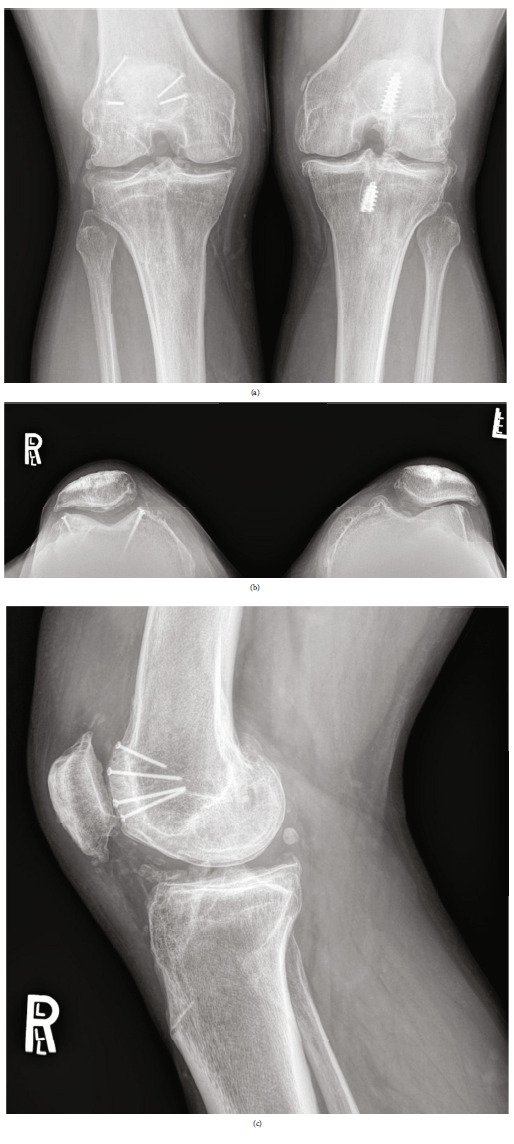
Four-year postoperative radiographs demonstrating excellent bone incorporation and maintained joint spaces with continued KL-2 grade on the right side. (a) Bilateral PA weightbearing. (b) Bilateral Merchant's view. (c) Lateral right knee.
